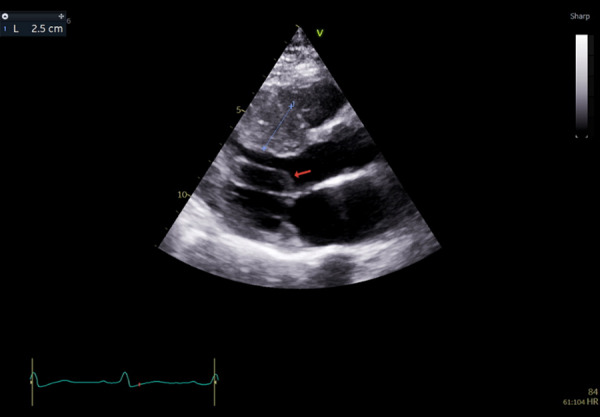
FIgure 2. Long Axis View Parasternal showing Systolic Anterior Motion (Arrow) sign, Hypertrophic Septum Measuring 2.5 cm (blue indicator)
